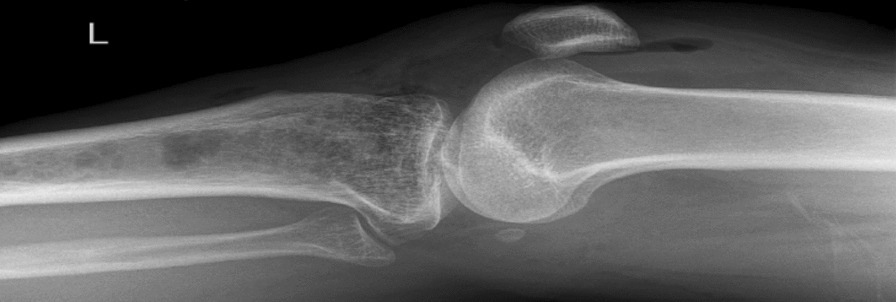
X-ray of the left knee shows osteolytic bone lesions of the tibia and gas in the suprapatellar pouch
